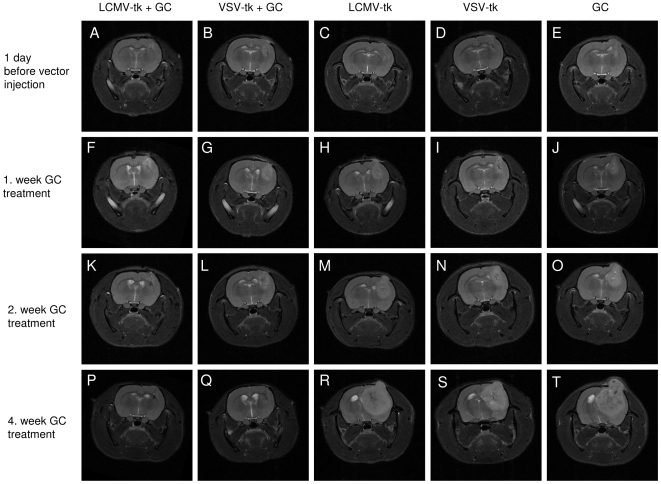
Tumors treated with lentiviral vectors and ganciclovir show complete remission on MRI. Representative three-dimensional MRI (T2 RARE). (A,F,K,P) Lentiviral LCMV-GP vectors with ganciclovir treatment. (B,G,L,Q) Lentiviral VSV-G vectors with ganciclovir treatment. (C,H,M,R) Lentiviral LCMV-GP vectors without ganciclovir treatment. (D,I,N,S) Lentiviral VSV-G vectors without ganciclovir treatment. (E,J,O,T) ganciclovir treatment only. Time points after tumor implantation: (A-E) 1 day before vector injection. (F-J) 1. week ganciclovir treatment. (K-O) 2. week ganciclovir treatment. (P-T) 4. week ganciclovir treatment.
